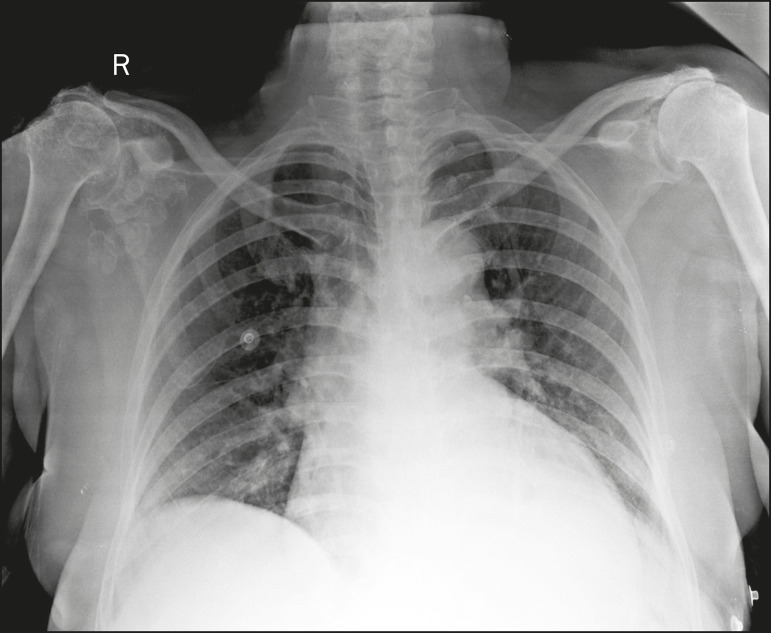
Hydroxyapatite-induced arthropathy in Milwaukee shoulder syndrome. Frontal X-ray of the chest showing the right shoulder with joint surface deformity, joint space reduction, and superior displacement of the humeral head with loss of the subacromial space (probably associated with a rotator cuff tear). Periarticular calcifications and intra-articular loose bodies can also be seen.
